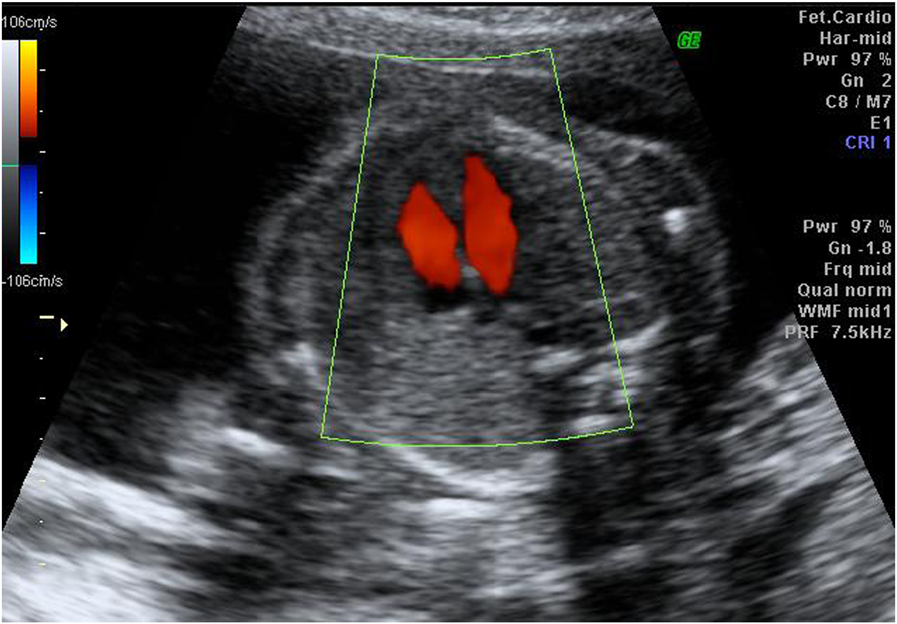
Color Doppler flow mapping.
